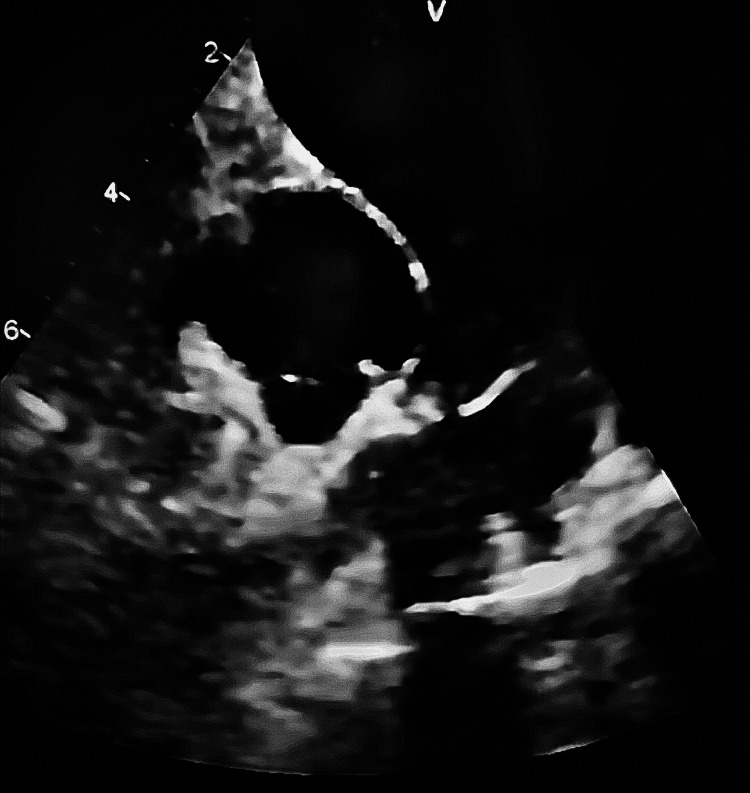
Postoperative echocardiographic view of the implanted pulmonary bioprosthetic valve. Postoperative echocardiographic image demonstrating the implanted pulmonary valve prosthesis with preserved structural appearance. This view allows anatomical assessment; however, detailed hemodynamic evaluation requires Doppler imaging.
